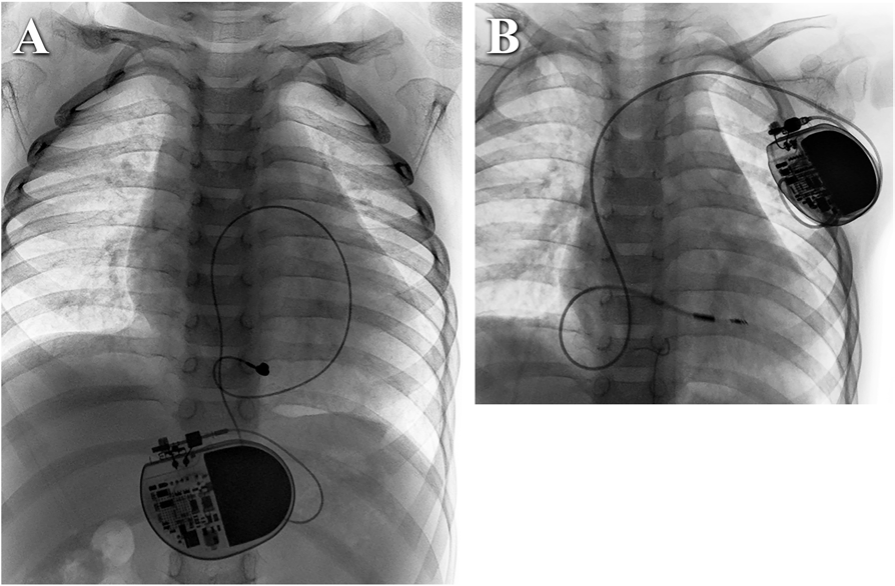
Chest X-ray imaging after epicardial and endocardial pacing. A The chest radiograph of an epicardial lead was implanted, and the pulse generator was buried beneath the rectus abdominis muscle. B The chest radiograph after endocardial pacing
